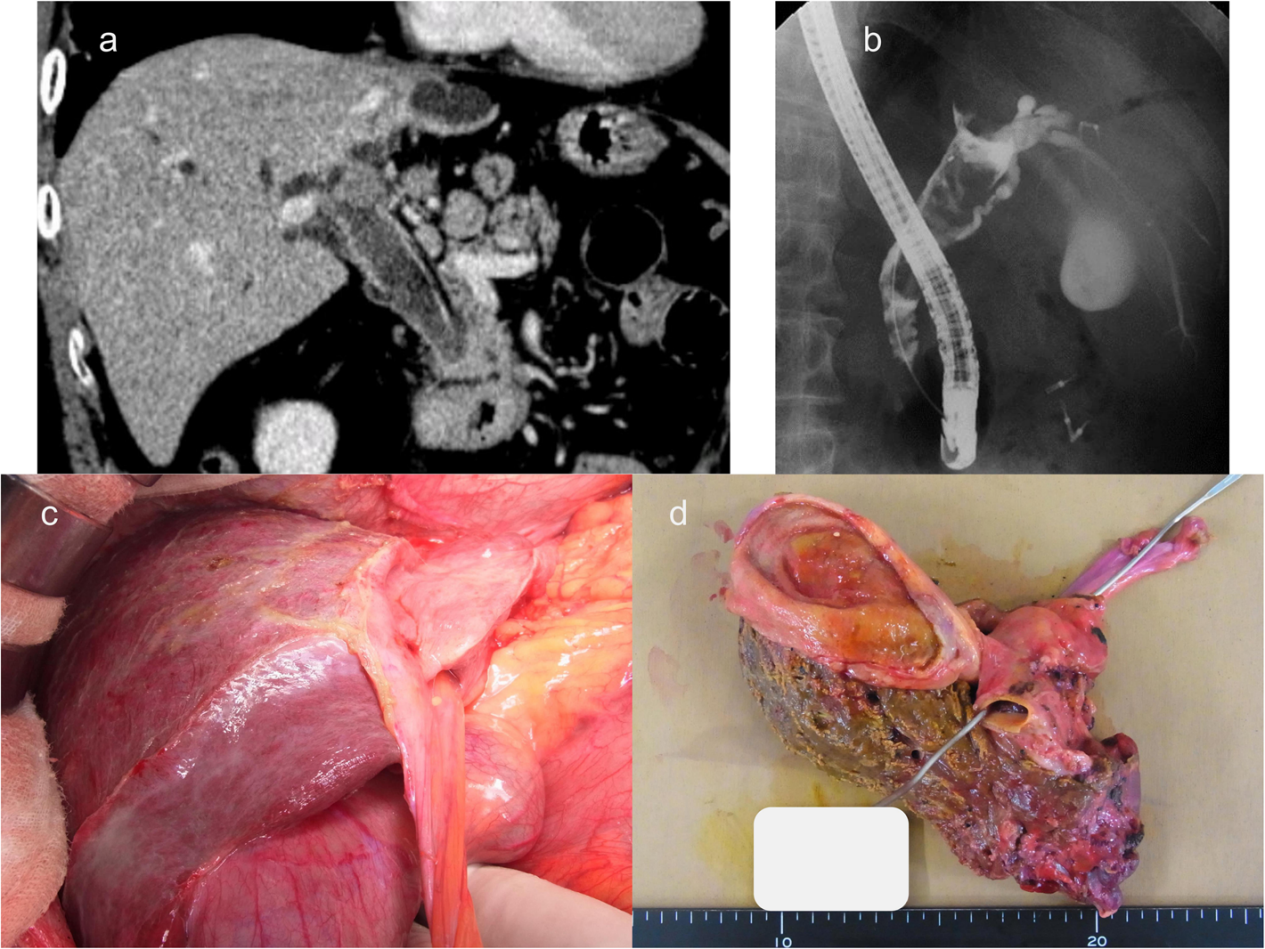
Contrast-enhanced computed tomography (CT) and endoscopic retrograde cholangiopancreatography (ERCP). a CT shows dilation of the intrahepatic and extrahepatic bile ducts, wall thickening of the common bile duct, and atrophy of the left liver lobe. b ERCP demonstrates a defect in the left intrahepatic and common bile ducts. Furthermore, a mass in the common bile duct is indicated. c Intraoperative findings showed atrophy of the left lobe. d Left hepatectomy, caudate lobectomy, and extrahepatic bile duct resection were performed
